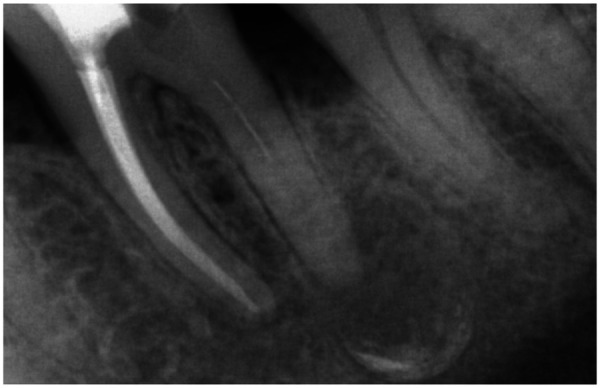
Right post-filling intraoral radiograph of tooth 36.
